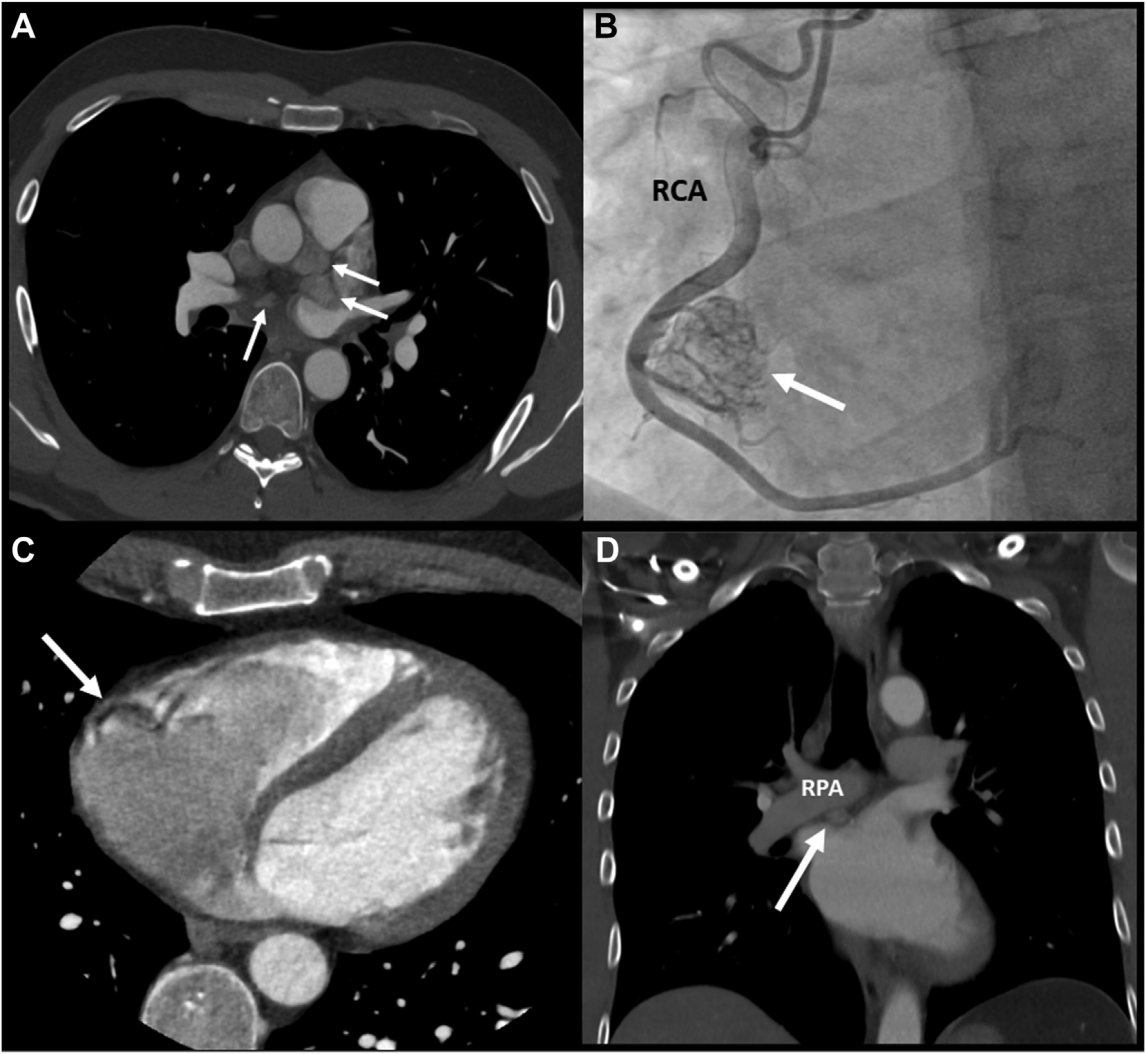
Preoperative imaging of the cardiac paragangliomas, which are indicated with a white arrows. (A) Transverse view of computed tomography (CT) scan demonstrating multiple paragangliomas with white arrows. (B) Angiogram of the RCA demonstrating the vascularization of the paranglioma adjacent to the RCA. (C) Transverse view of CT scan showing the paraganglioma near the RCA. (D) Frontal view of CT scan showing the paraganglioma between the RPA and the left atrium. LCA, left coronary artery; RCA, right coronary artery; RPA, right pulmonary artery.
